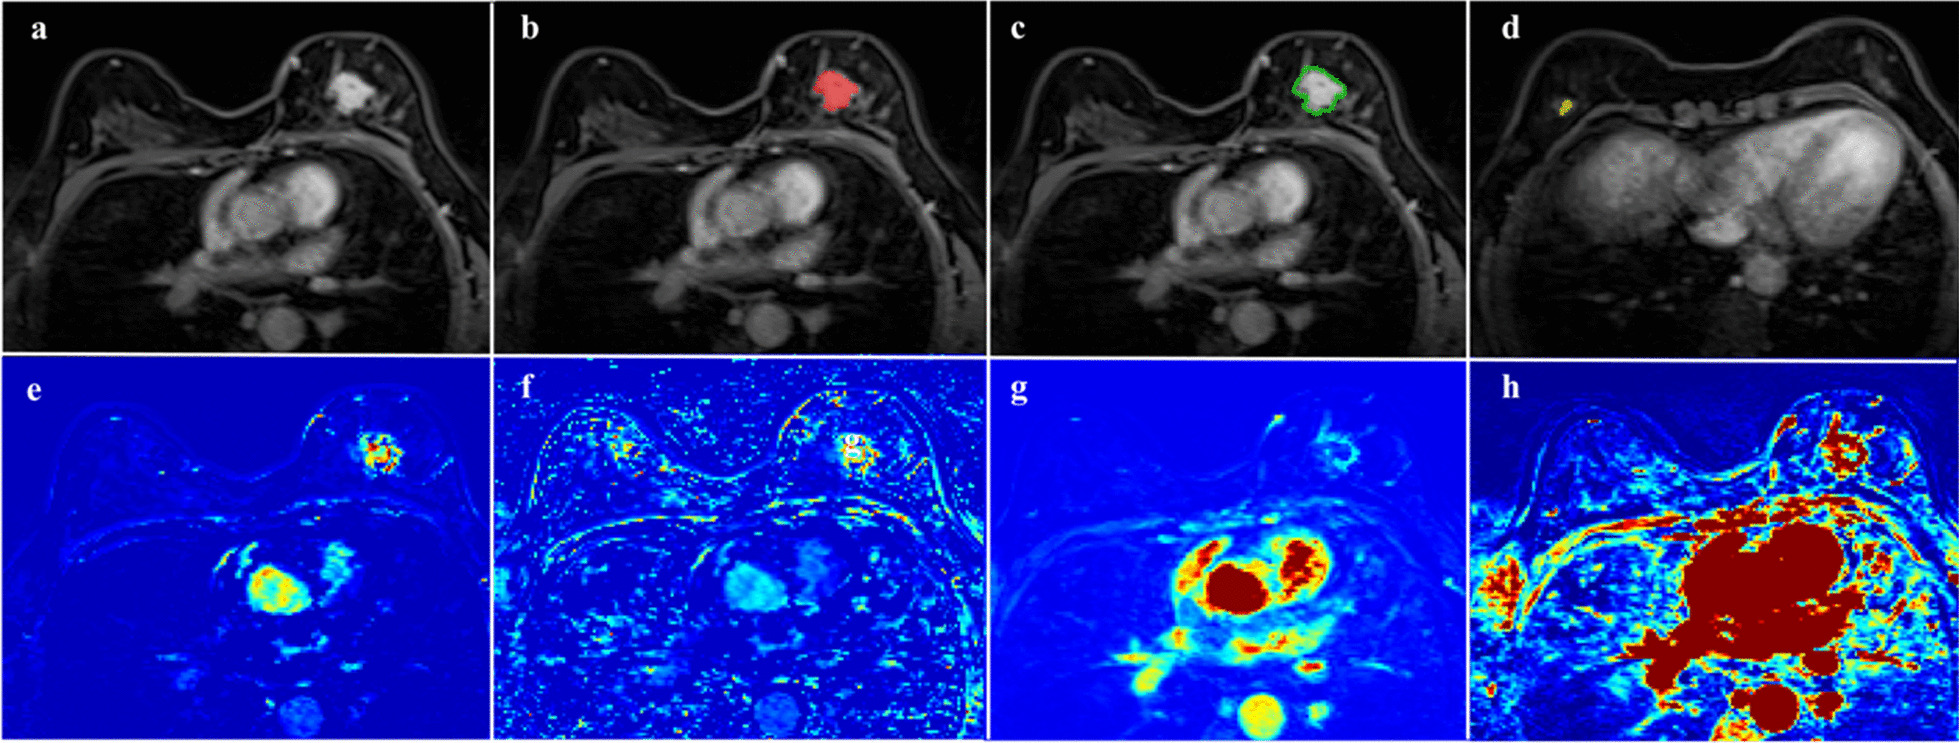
A 51-year-old female with BI-RADS 4c. A mass was clearly identified in the left breast on enhanced T1-weighted imaging (a–d), K_ep_ (e), V_p_ (f), K^trans^ (g), and K^trans^ map (h) from high-temporal-resolution DCE-MRI. All ROIs were manually drawn to cover the intralesional area (b, red color), perilesional area (c, green color), and background parenchymal enhancement area (d, yellow color). The pathological result was breast invasive ductal carcinoma (grade III)
